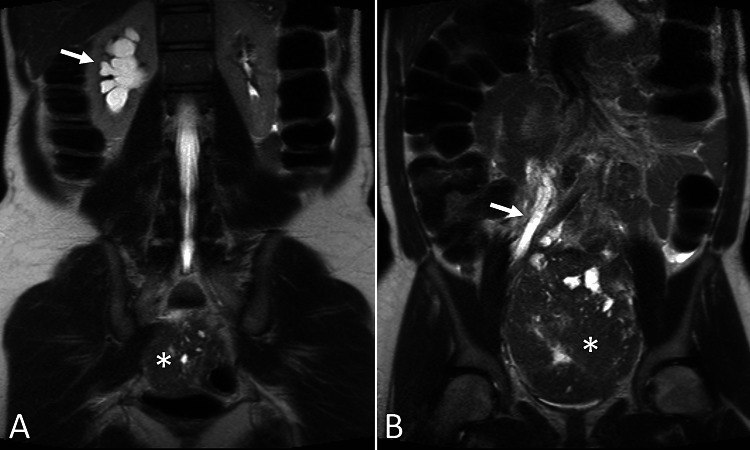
T2 weighted pelvic MRI. Coronal T2 weighted pelvic MRI demonstrates the pelvic mass with cystic components (asterisks). There is right-sided hydronephrosis (arrow in A) and hydroureter (arrow in B) due to distal ureteral obstruction by the mass.
